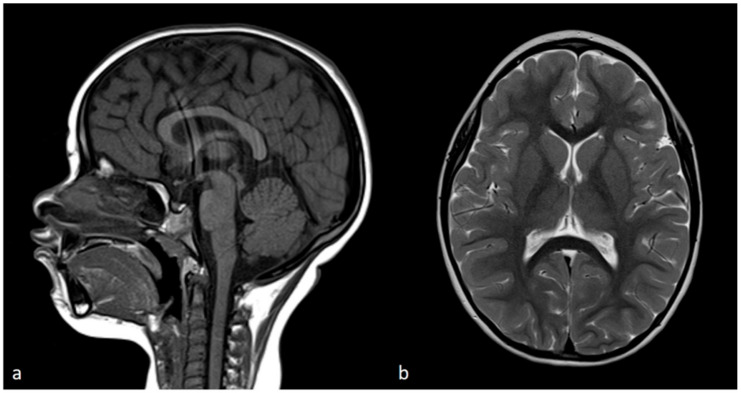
Brain MRI. (a) Sagittal T1-weighted image showing complete formation of the corpus callosum and normal shape and volume of cerebellar vermis and brainstem. (b) Axial T2-weighted image displays adequate white matter myelinization and normal basal ganglia volumes.
